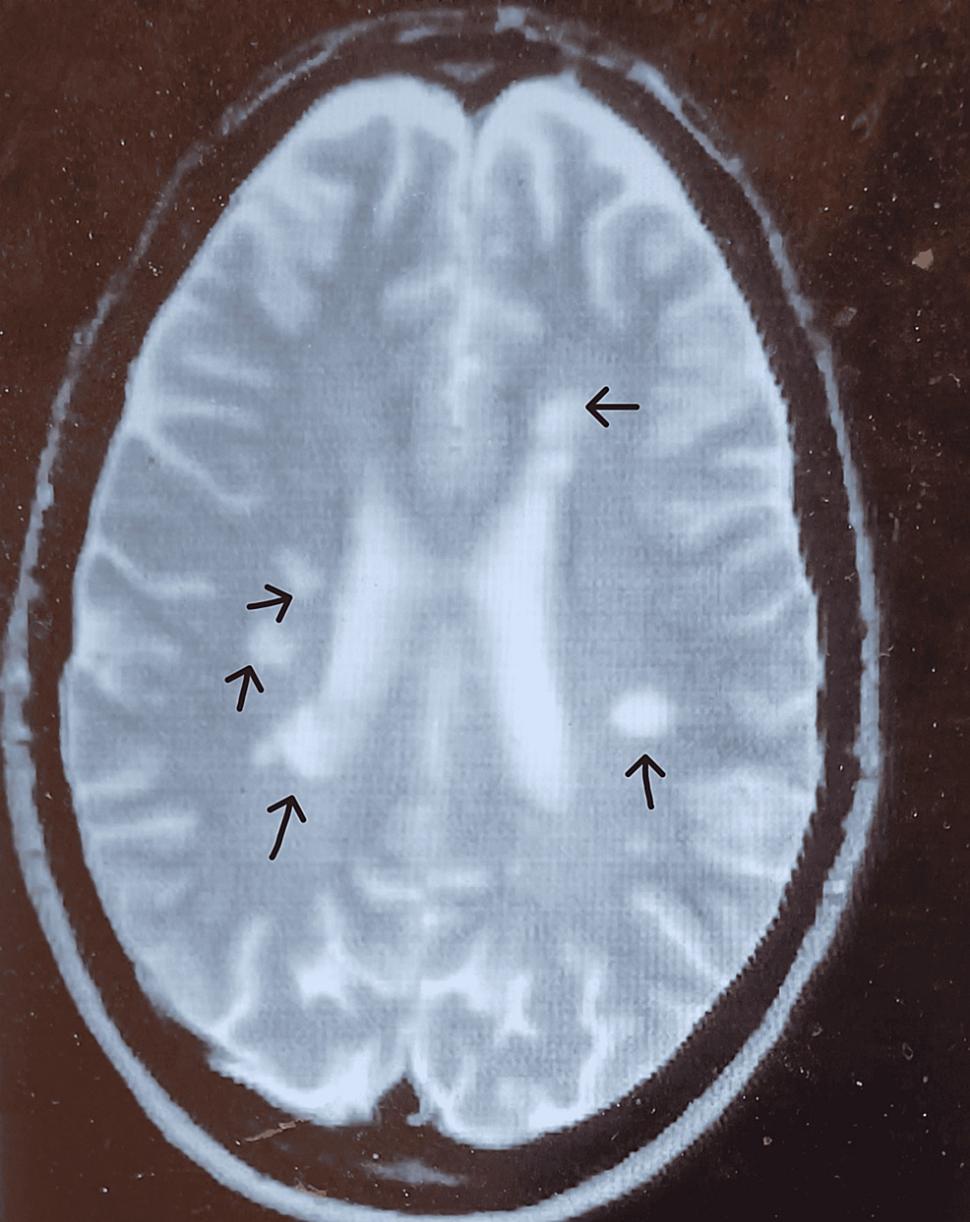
T2 MRI with arrows indicating the location of prior identified MS lesions. Arrows: the multiple MS lesions of the patient in the case report on MRI imaging. The lesions appear chronic, paraventricular, multiple, and bilateral - these characteristics have been linked with psychosis in MS.
